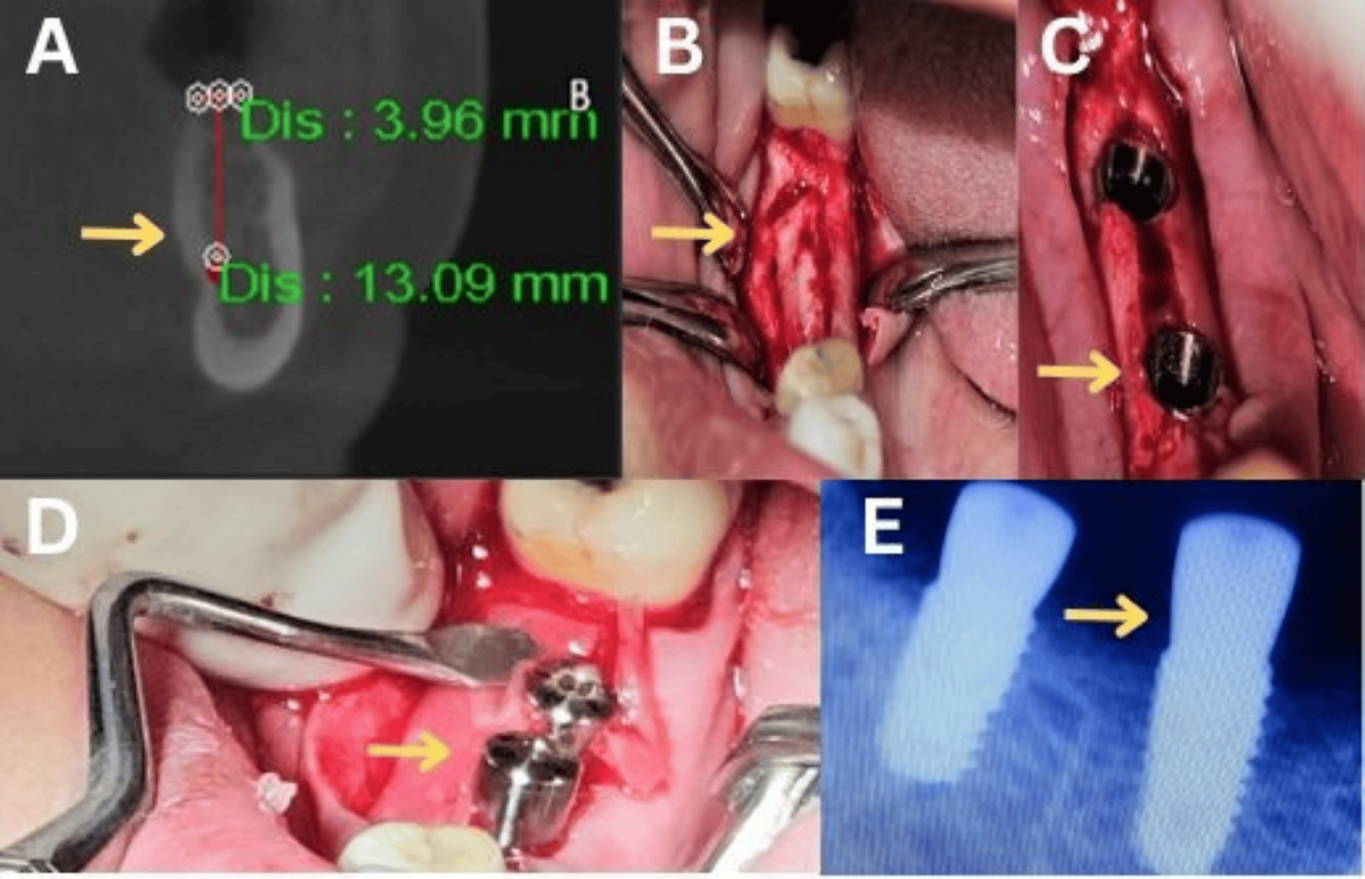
Conventional ridge split technique. (A) Pre-operative cone-beam computed tomographic measurements of the mandible at the lower right first molar region (yellow arrow). (B) Horizontal and vertical incisions in the alveolar crest (yellow arrow). (C) Implant placement (yellow arrow). (D) Healing abutment placement (yellow arrow). (E) Post-implant intraoral periapical radiograph of the lower right first and second molars (yellow arrow). This figure represents intraoral pictures of a patient from the study, and was published with the patient's consent.
